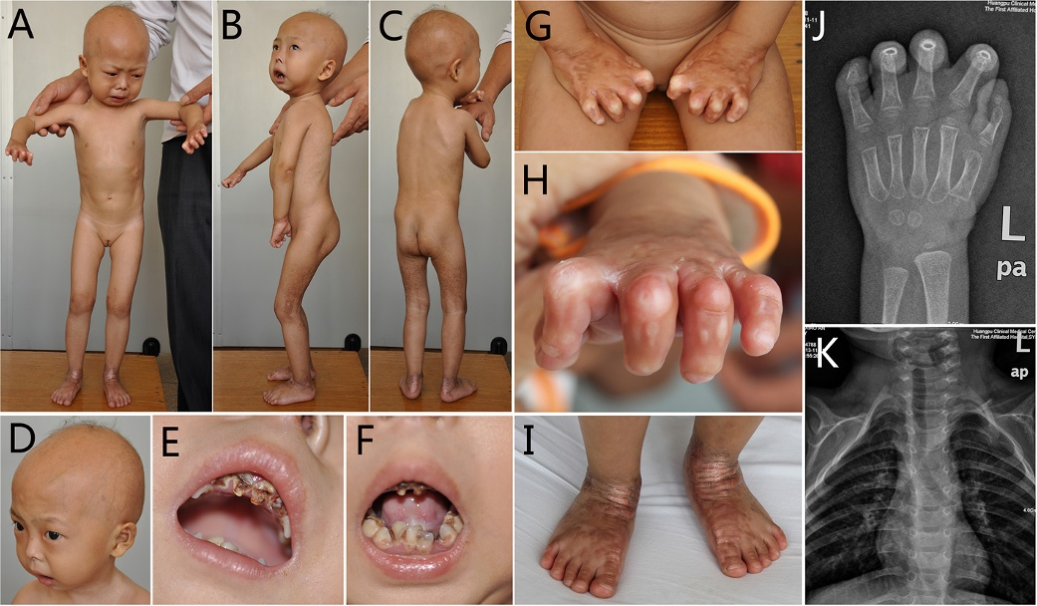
Patient 2 shows a senile appearance with hyper- or hypo-pigmentation, sclerodermatous changes and thin skin on the lower abdomen, buttocks, elbows and the lower extremities, lipodystrophy of the gluteal region and extremities (A-C); sparse scalp hair with easily visible veins (D); irregular and carious teeth and crowded teeth on the mandible (E,F); severe contractures at the interphalangeal joints and the hands with abnormal posture (G,H), and coarse and thickened skin around the ankles and on the back of feet in symmetry (I). X-ray findings of hand show flexion deformity of fingers of hands and delayed bone age (J). Radiological changes in chest reveal pyriform thorax , absence of right clavicle, and absence of mid-lateral part with thin interior part of left clavicle, and thin posterior parts of the 2^nd^ and 3^rd^ ribs on both sides with disconnection of posterior parts of the 3^rd^ rib on left side and the 2^nd^ and 3^rd^ on the right (K).
